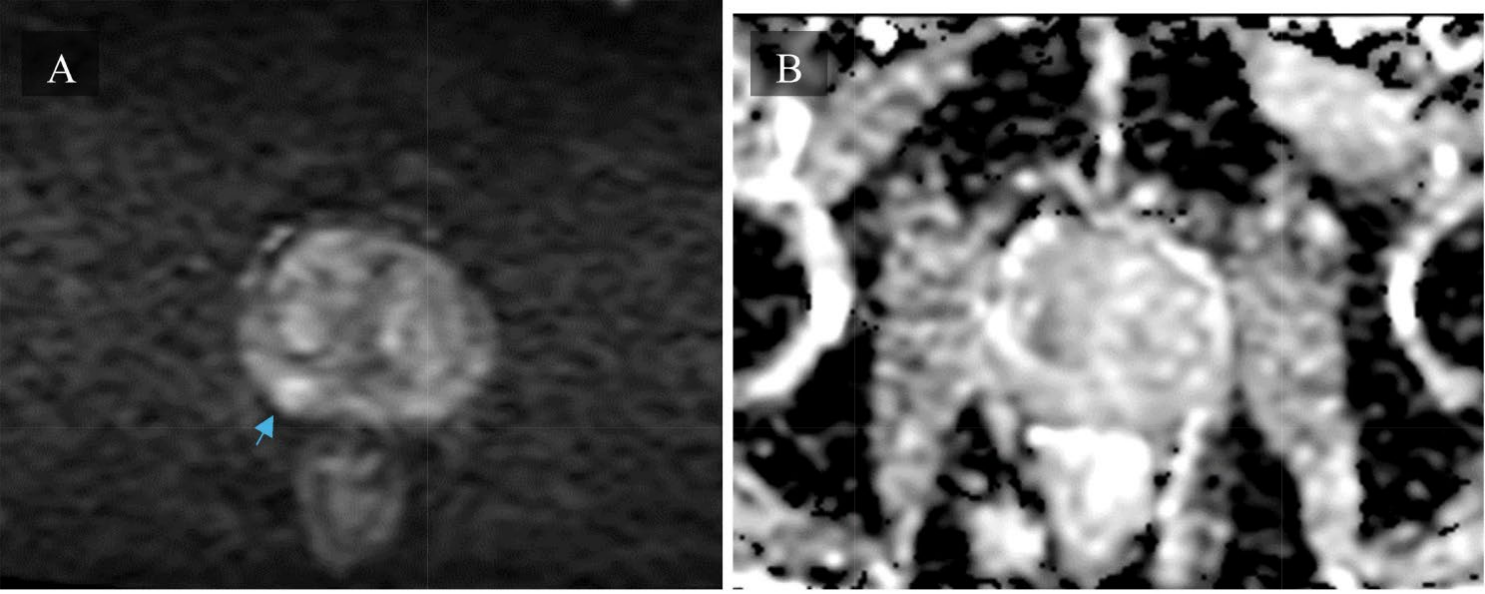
Axial DWI (A) & ADC (B) showing heterogenous nodular restricted diffusion throughout transition zone as well as low grade mass in right peripheral zone (blue arrow). DWI: Diffusion-weighted imaging. ADC: Apparent Diffusion Coefficient
